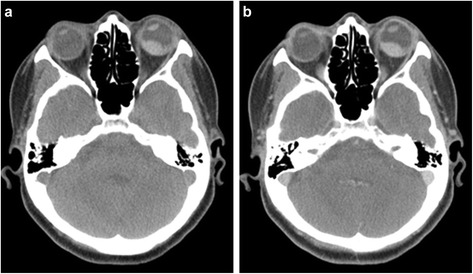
Noncontrast CT detected a high attenuation mass (16 × 11 mm) in the left choroid plexus (a) that showed enhancement after contrast administration (b)
